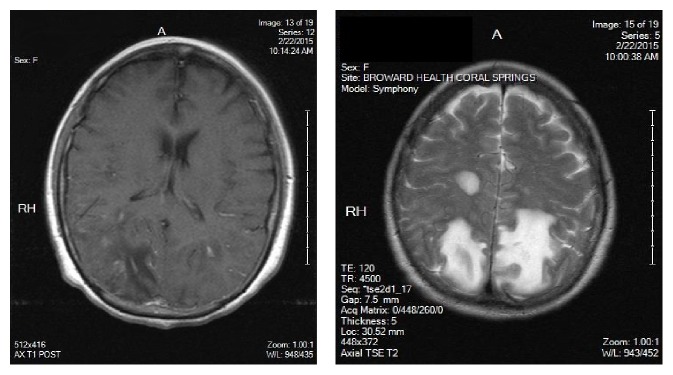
Brain MRI on admission: multiple enhancing masses in the bilateral occipital and posterior parietal lobes on T1 and T2. Enhancement in the right centrum semiovale is also visualized.
